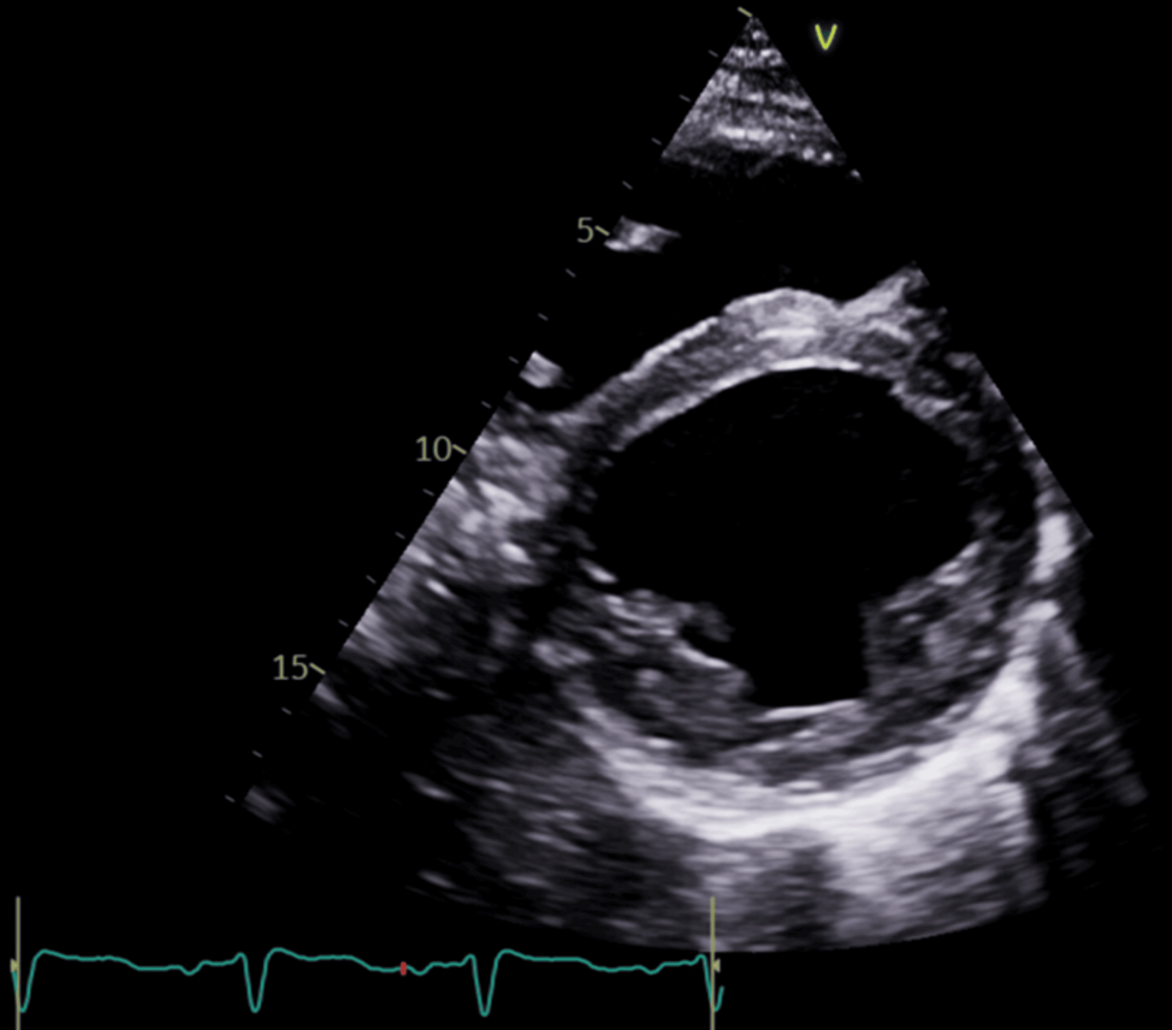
Parasternal short-axis view echocardiogram showing biventricular enlargement with reduced systolic function (EF 15%). EF: ejection fraction
